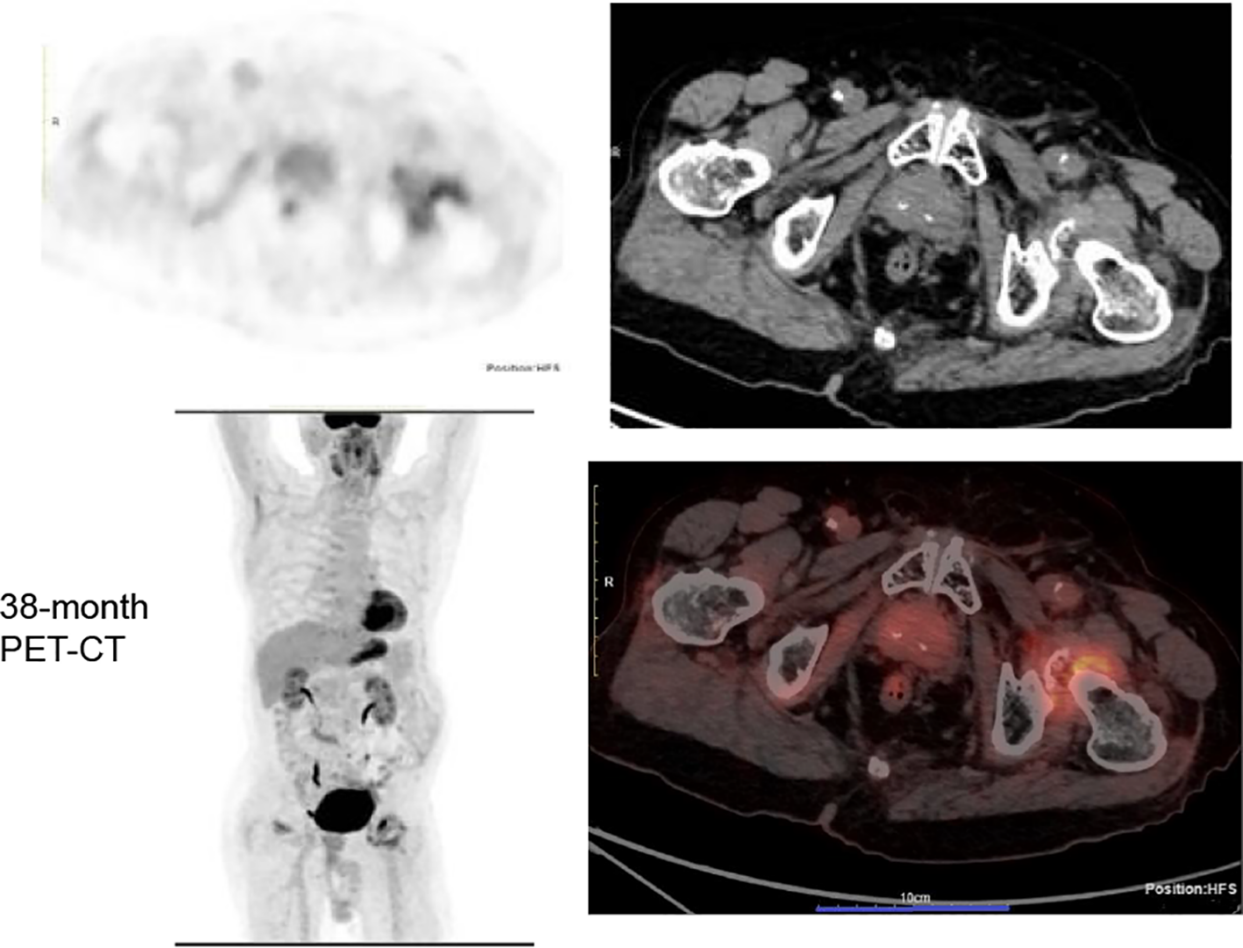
38-month followed-up PET-CT.No evidence of local tumor recurrence at the primary surgical site and no FDG-avid lymph nodes in the left inguinal region by PET/CT scan.
